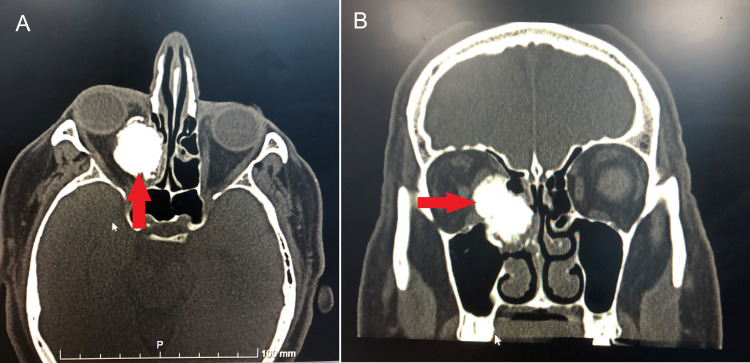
Preoperative computed tomography (CT) scan showing the lesion in cross-sectional view (A) and coronal view (B)
